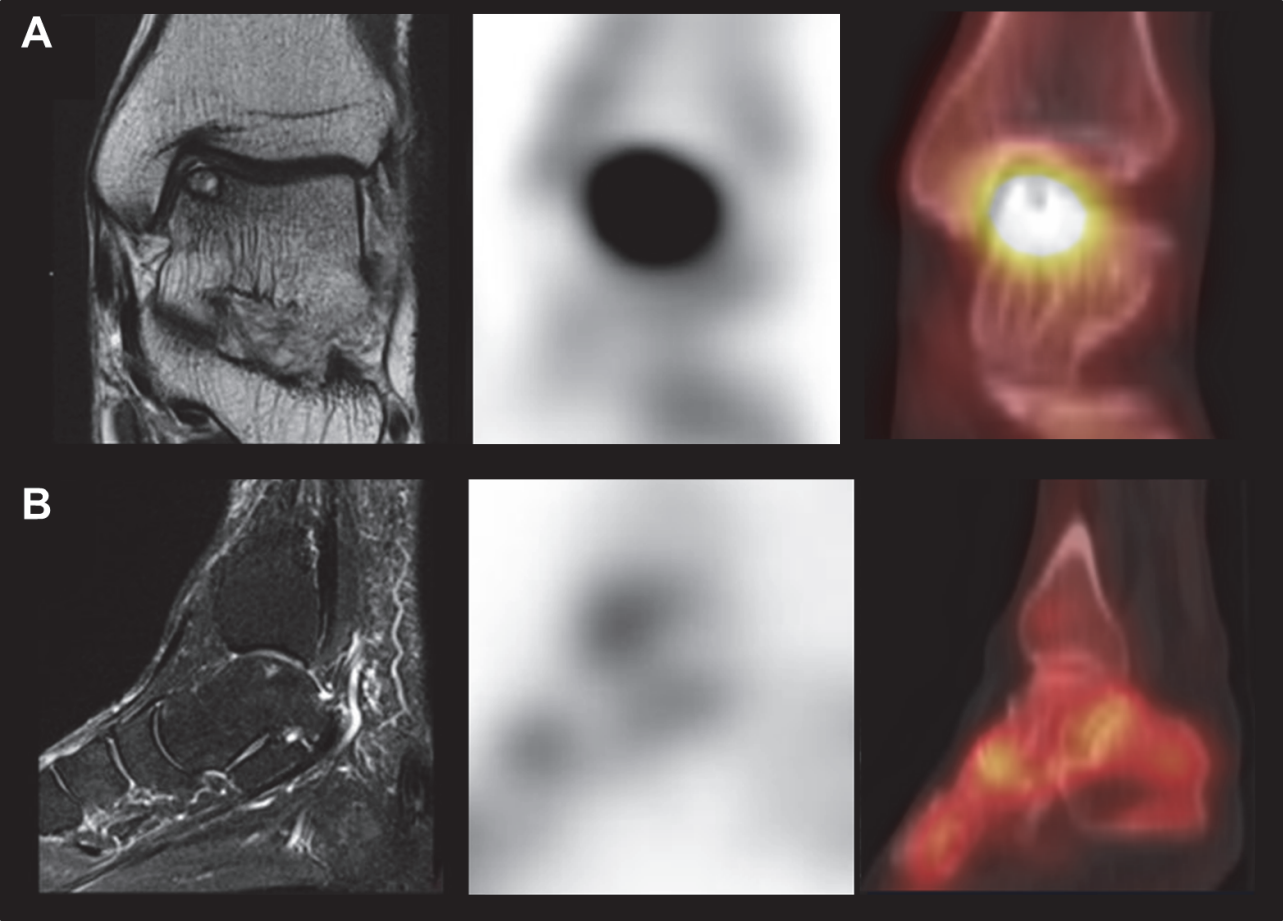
Images of representative cases. (A) Axial MRI (left) of a19-year-old woman revealed an osteochondral lesion in the right medial talar dome area. SPECT/CT (middle and right) also exhibited focal intense uptake in the same area, concordant with MRI. The lesion was diagnosed with symptomatic osteochondral lesion of talus, and her ankle pain improved after arthroscopic multiple drilling of the talus. (B) Sagittal fat-saturated MRI (left) of a 65-year-old woman with posterior heel pain revealed an osteochondral lesion in the right medial talar dome area, whereas SPECT/CT showed no abnormal uptake in the same area. The pain improved after surgical removal of a right calcaneal bony fragment, and the talar dome lesion was determined to be asymptomatic.
